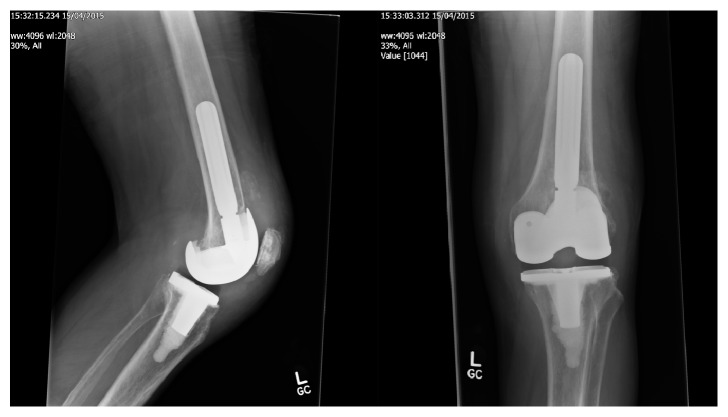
Postoperative radiograph of 2nd stage revision with nickel-free prosthesis.
